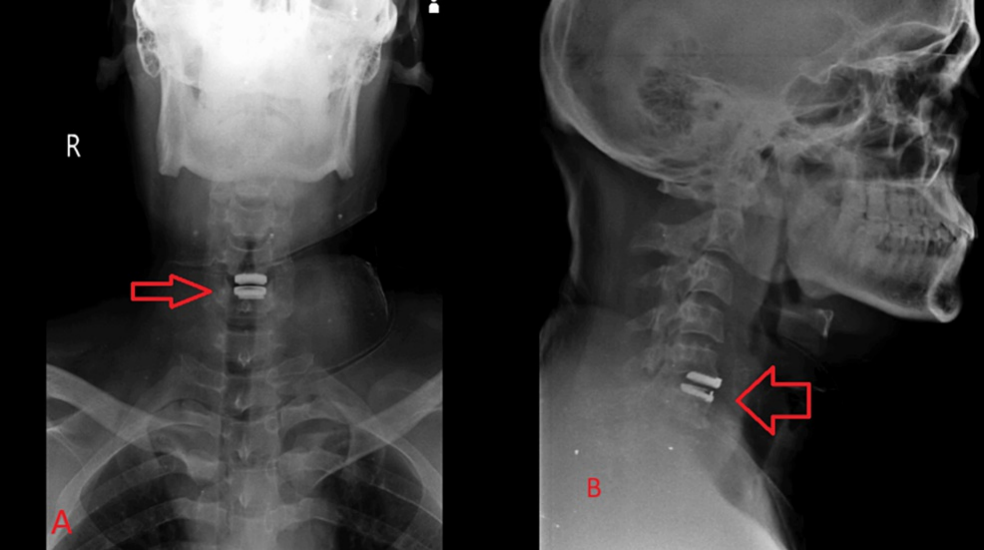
Radiographs ((A) anteroposterior and (B) lateral views) on immediate postoperative day 2 showing artificial disc at the level of C5-C6 with good alignment and placement (arrows)
